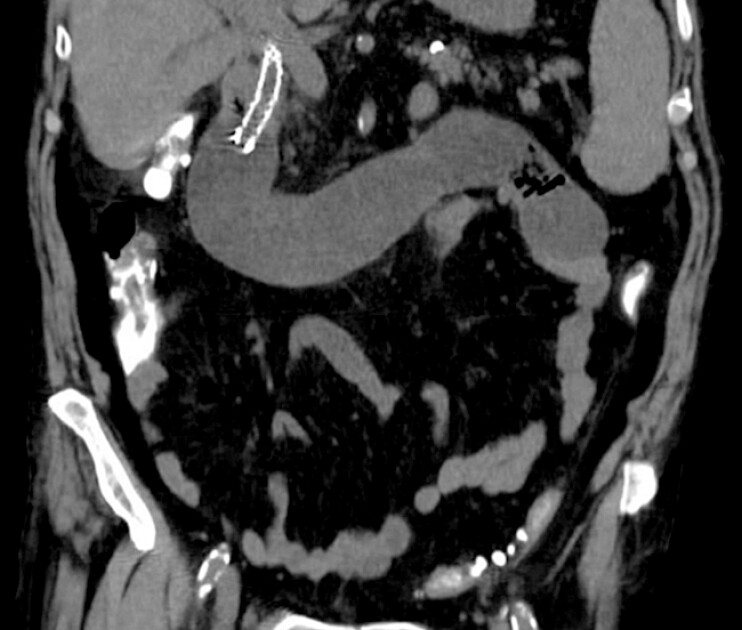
A coronal CT section demonstrating afferent loop obstruction with upstream small bowel dilation. CT, computed tomography.
